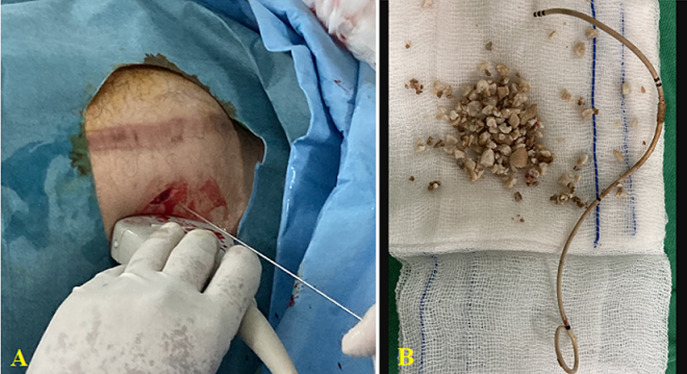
A) percutaneous punctures to the kidney with ultrasound guide; B) stones and DJ stents were successfully removed
